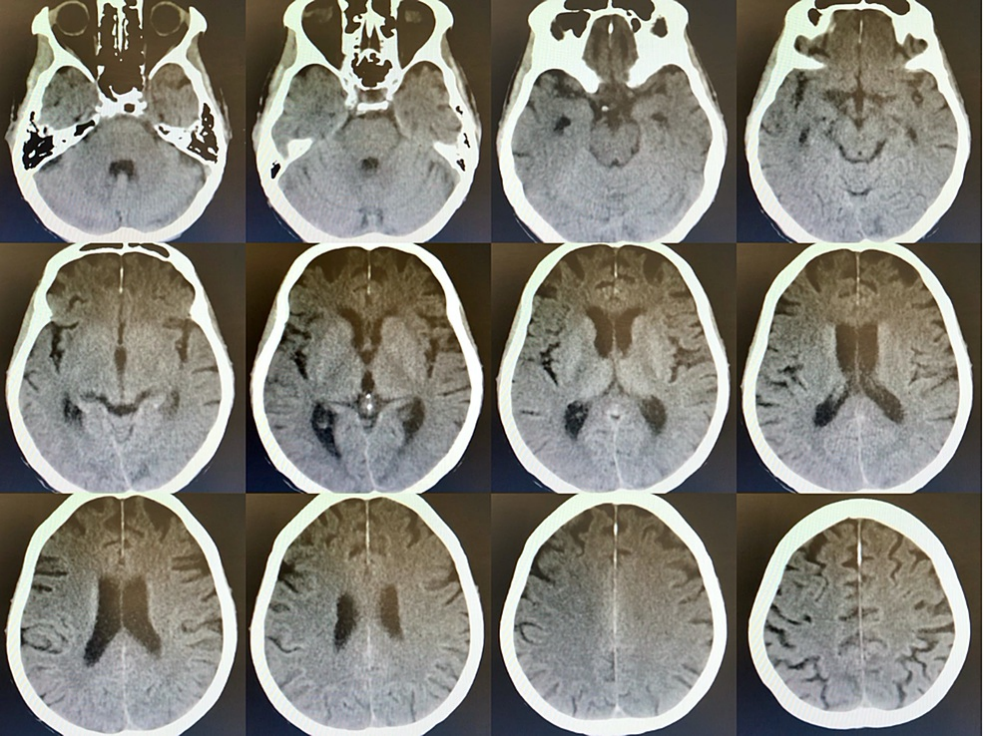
Non-contrast CT brain (after the seizure episode following the first hemodialysis) did not show any hemorrhage, infarct, and space-occupying lesion
